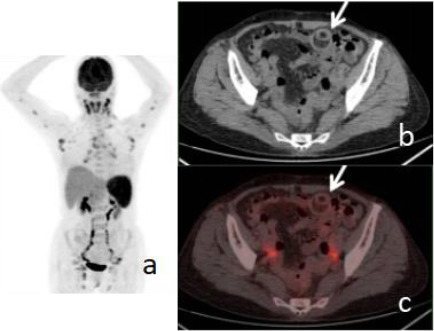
18F-FDG PET/CT of Case 1 showing intussusception due to an ileal lipoma. Multiple lymphadenopathy and splenomegaly with FDG avidity were shown in PET/MIP (a). Double sign (arrows) shown in CT (b) and fusion image (c).
